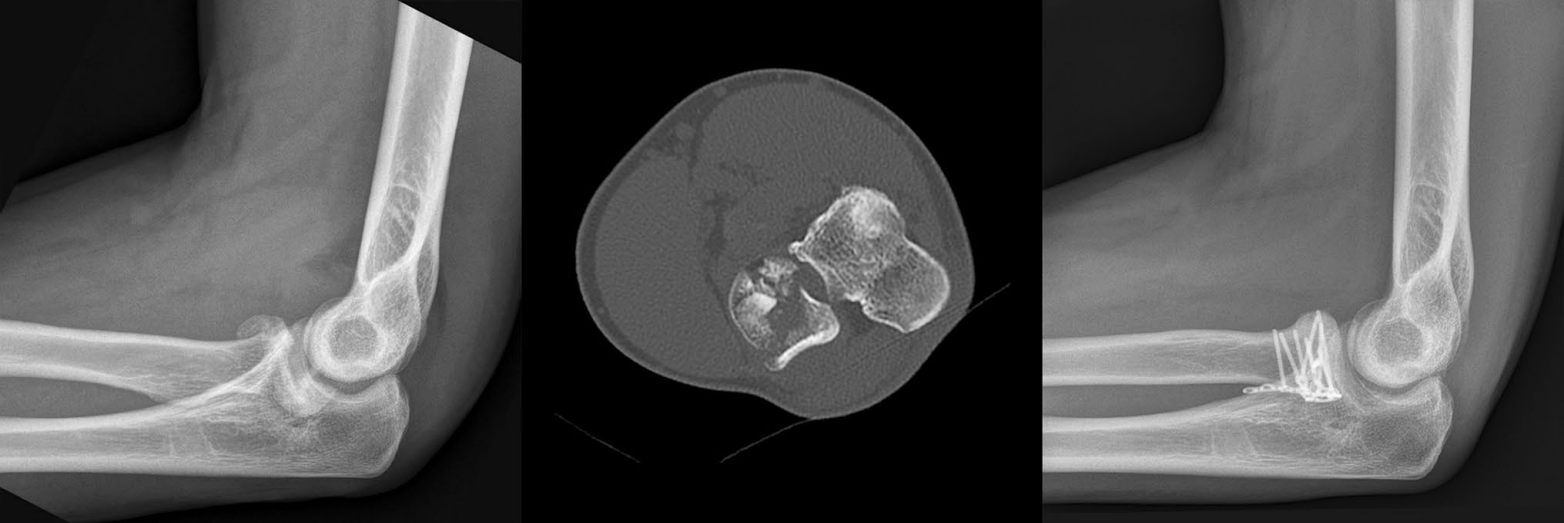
Preoperative radiograph, axial CT scan and postoperative radiograph of a 4-part radial head fracture treated by ORIF with plates
